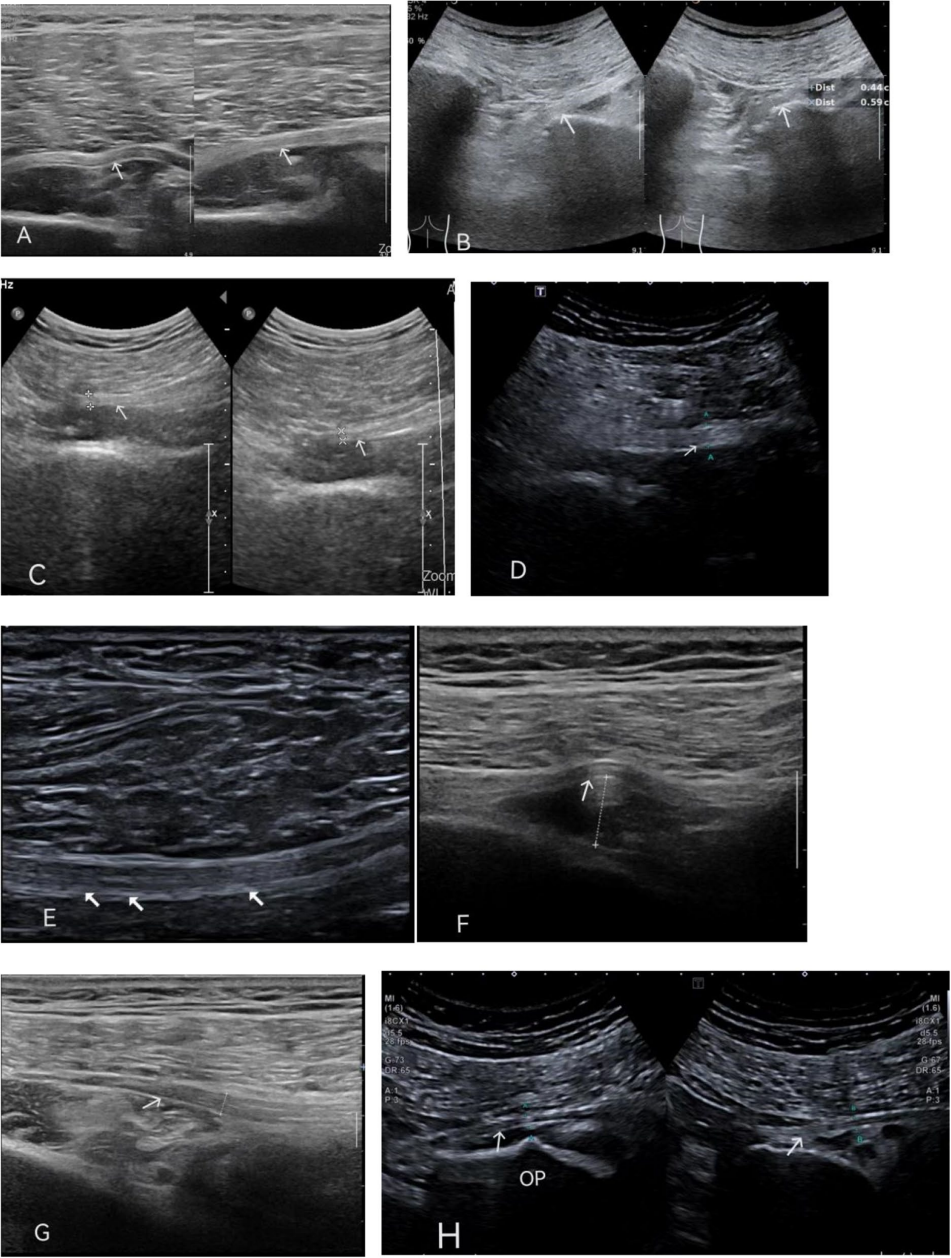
Preoperative ultrasound of various morphological changes in the sciatic nerve (SN) (the SN are marked with white arrows). A SN distorted with inhomogeneous border. B SN thickened (left). C SN thinned (right). D Gluteal muscle echo inhomogeneous with unclear SN border. E Thickness of SN bundles and not uniform. F Superior gemellus muscle oedema and SN thinning. G Synovial hyperplasia. H Osteophyte (OP)
